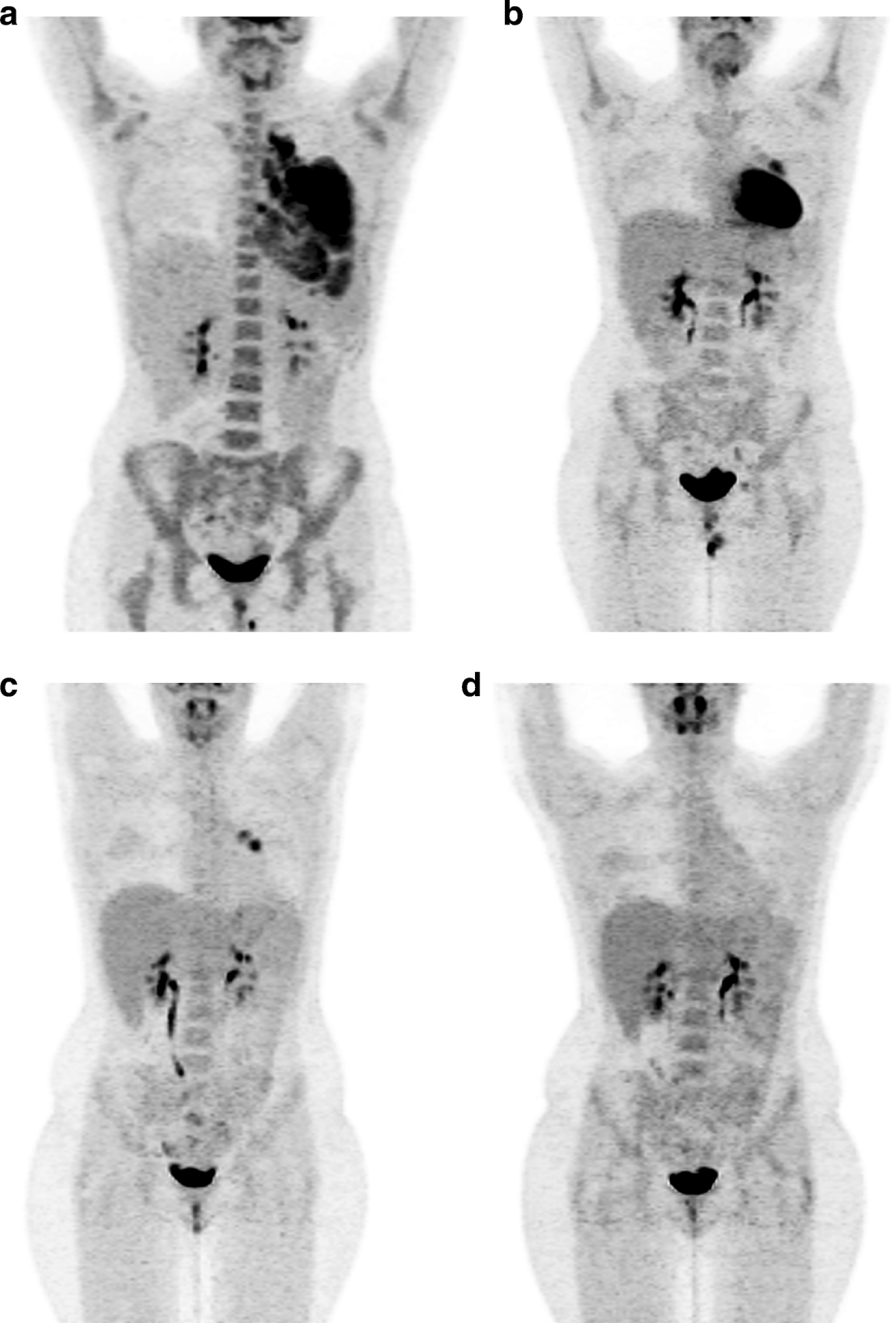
A 25-year-old woman with Hodgkin lymphoma. Maximum intensity projections of FDG PET. a The initial staging PET image reveals stage II disease. b The PET image after six cycles of chemotherapy (ABVD) shows PET-positive residual uptake at the left side of the mediastinum (Deauville score 5). c The PET image after involved field radiotherapy now demonstrates an additional focus of uptake (physiological heart uptake is suppressed on this scan). Biopsy revealed posttreatment changes only. d The interval PET image 12 months later shows resolution of uptake
